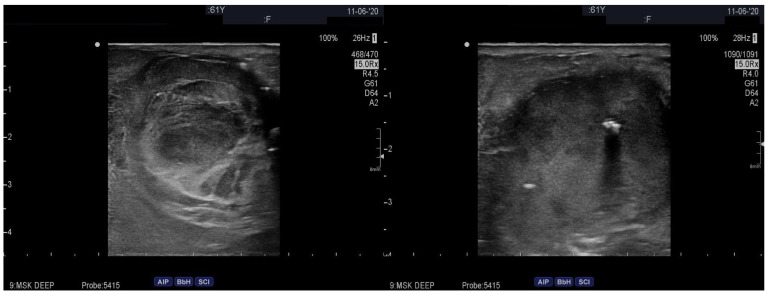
Great saphenous vein aneurysm on initial ultrasound examination.
